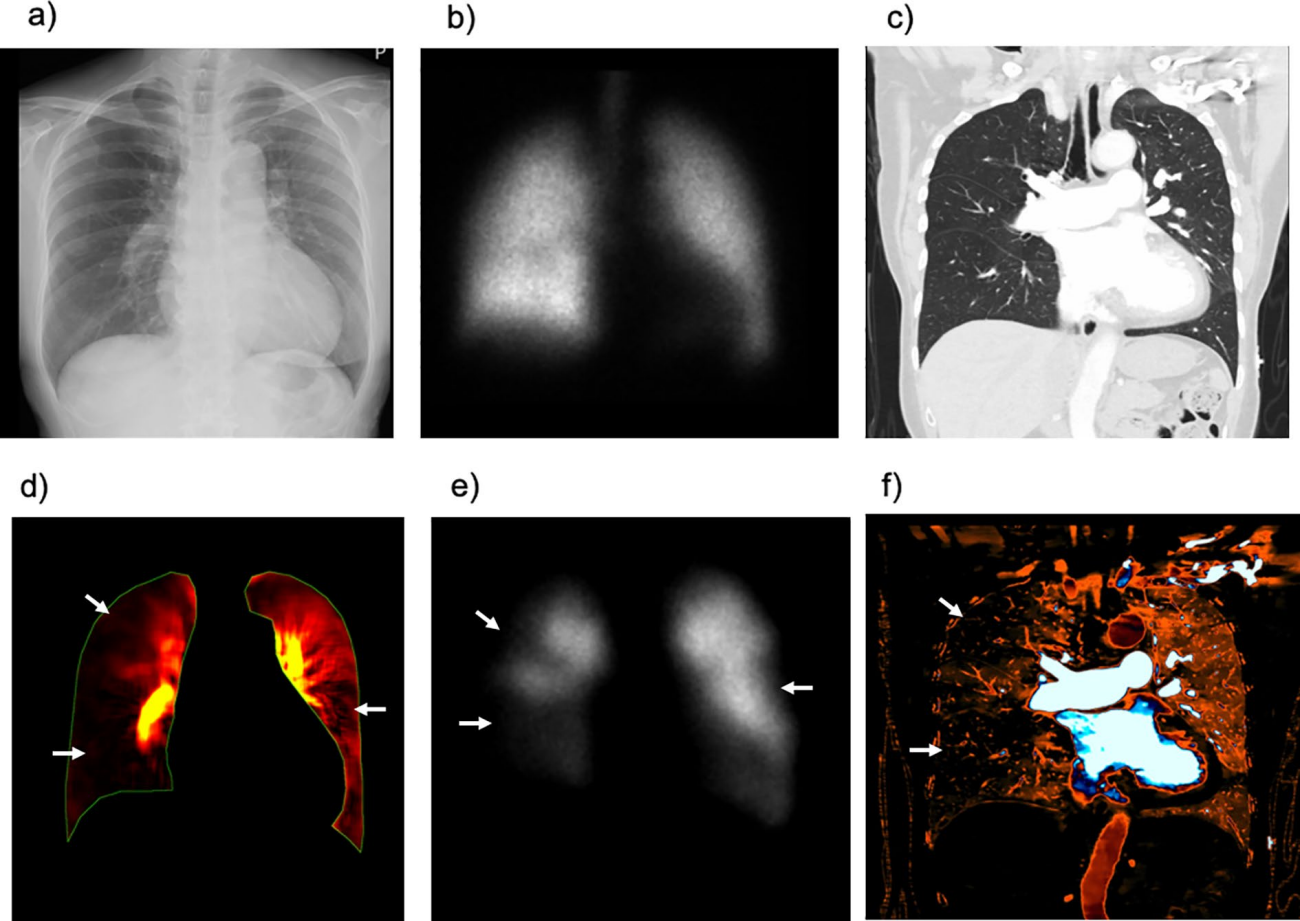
Images of a 67-year-old woman with chronic thromboembolic pulmonary hypertension. Normal lung findings are shown in the chest radiograph (a), anterior planar ventilation scintigraphy (b), and coronal view of the chest computed tomography (CT) (c), whereas wedge-shaped perfusion abnormalities are observed in the perfusion image on dynamic chest radiography (d), anterior planar perfusion scintigraphy (e), and iodine map created from CT pulmonary angiography (f)
